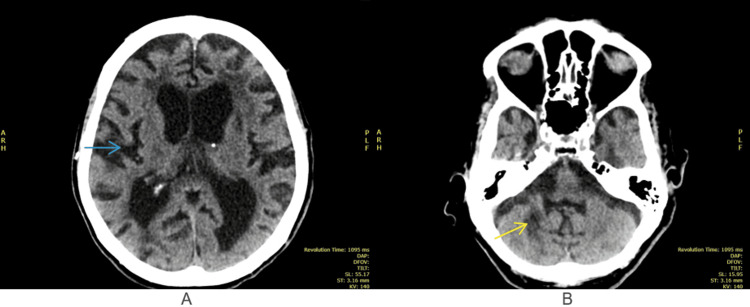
CT of the brain demonstrating (A) advanced degenerative atrophy (blue arrow), and (B) old right cerebellar cerebrovascular accident (yellow arrow).
